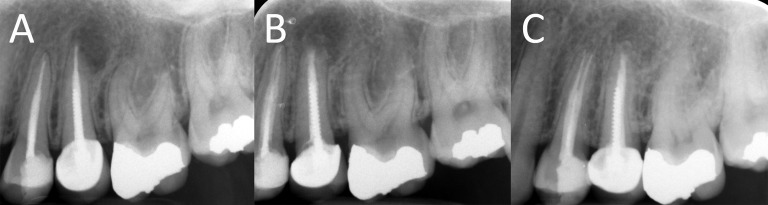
A) A maxillary premolar with moderate apical lesion (case No. 10); B) immediate postoperative radiograph; C) the tooth 15 months after root-end surgery shows complete periradicular healing
